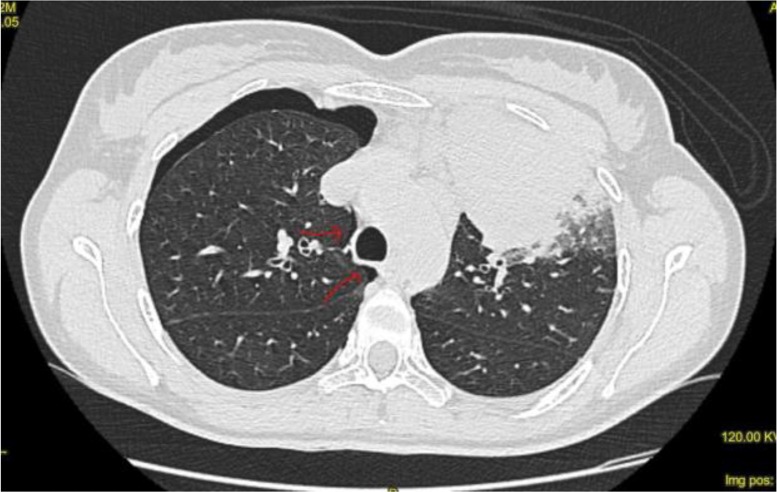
Computed tomography of thorax: pneumothorax involving the right side and slight pneumomediastinum (shown by the arrows)
